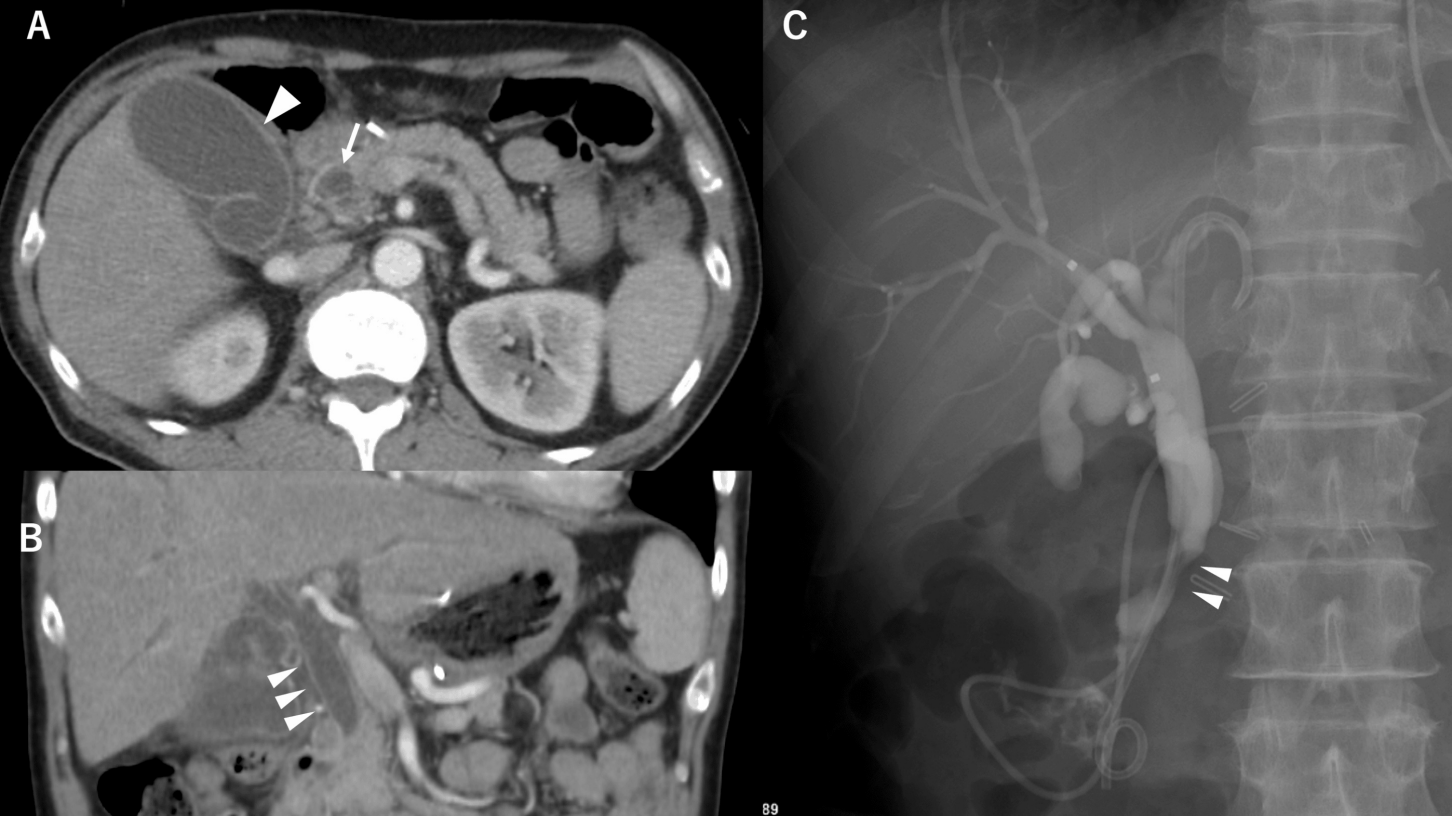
Clinical imaging findings at the time of immune-related adverse event cholangitis (A) Axial contrast-enhanced computed tomography (CECT) reveals the common bile duct (CBD) dilation (arrow) and diffuse gallbladder wall thickening (arrowhead). (B) Coronal CECT reveals the CBD dilation of up to 11 mm and diffuse CBD wall thickening (arrowheads). (C) Endoscopic retrograde cholangiopancreatography shows biliary tract dilation localized to the extrahepatic lesion with distal narrowing (arrowheads) and no obstruction.
